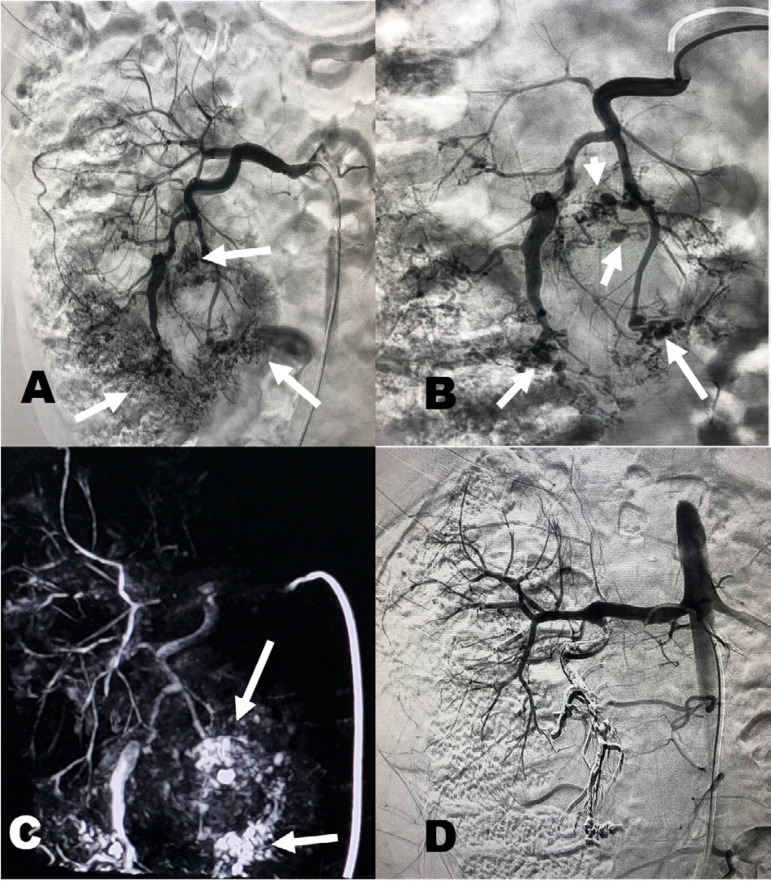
A: Arterial phase evidenced multiple saccular dilatations (aneurysms) within the inferior interlobar branches of the right renal artery (white arrow). B: Selective arteriography of the lower pole branch with multiple saccular dilations (White arrow). C: Cone beam CT was performed to assess the remaining kidney segments. In the reconstruction, a suspicious area of active bleeding was identified in the lower pole (white arrow). D: Superselective embolization of microaneurysms with Onix-18 and control arteriography with devascularization of the lower pole branches (white arrow).
